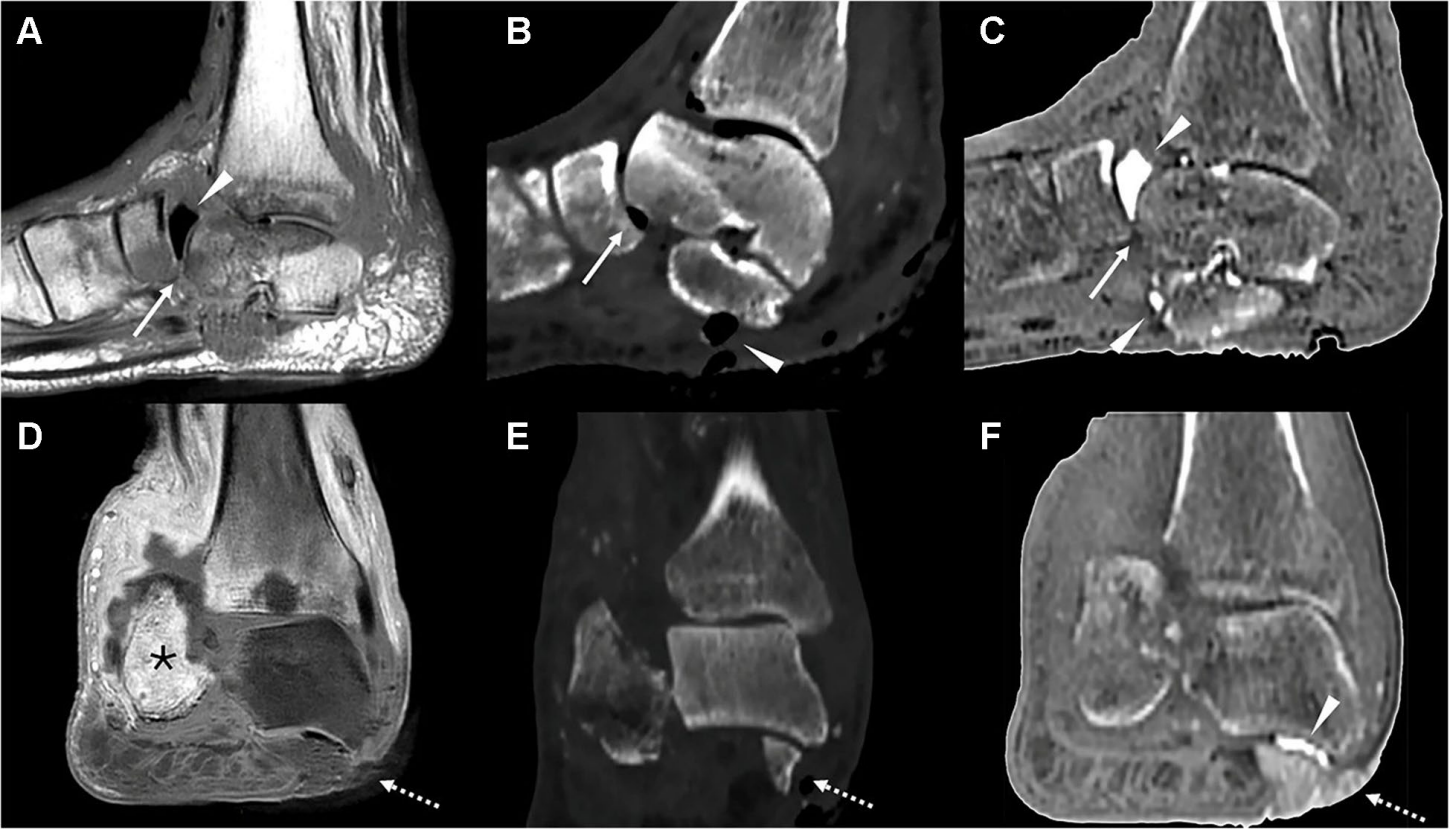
Late-stage Charcot foot with superimposed osteomyelitis in a 74-year-old male. Sagittal T1 (A), sagittal CT multiplanar reconstruction (B), sagittal ZTE reconstruction (C), coronal T1 post-contrast (D), coronal CT multiplanar reconstruction (E), and coronal ZTE reconstruction (F) images demonstrate severe hindfoot destruction with calcaneal fragmentation and erosion, talonavicular subluxation (arrow in A, B and C) and subtalar dislocation, soft tissue pneumatosis (arrowheads in A, B, C and F), and a hindfoot ulcer with exposure of the talus (dashed arrow in D, E and F). Note the diffuse bone contrast enhancement, particularly involving the calcaneus, indicative of osteomyelitis (*), as well as the enhancement of adjacent soft tissues, consistent with cellulitis
